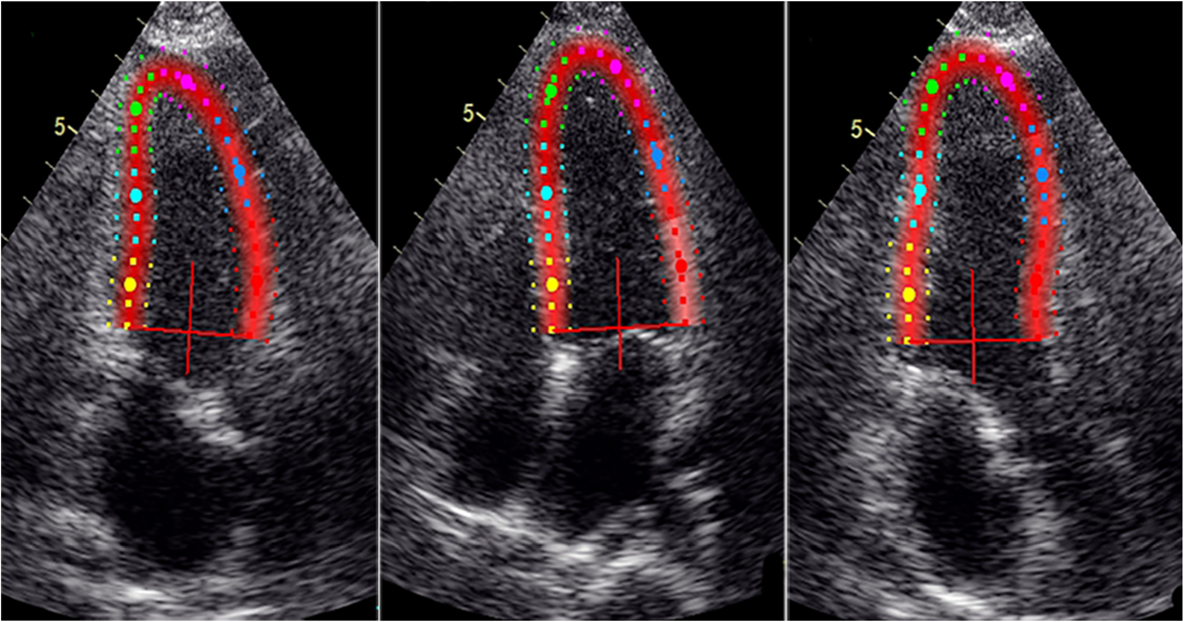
Multiple-dotted lines in three myocardial layers at the three parasternal long-axis scans. The endocardial borders are delineated in the end-systolic frame of the images at the apical four-chamber, two-chamber, and long-axis planes. Subsequently, the myocardial wall is automatically defined with multiple chains of nodes for allowing assessment of longitudinal endocardial, mid-myocardial and epicardial strains
